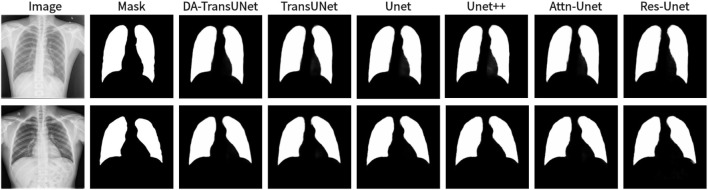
Comparison of qualitative results between DA-TransUNet and existing models on the task of segmenting Chest X-ray Masks and Labels X-ray datasets.
